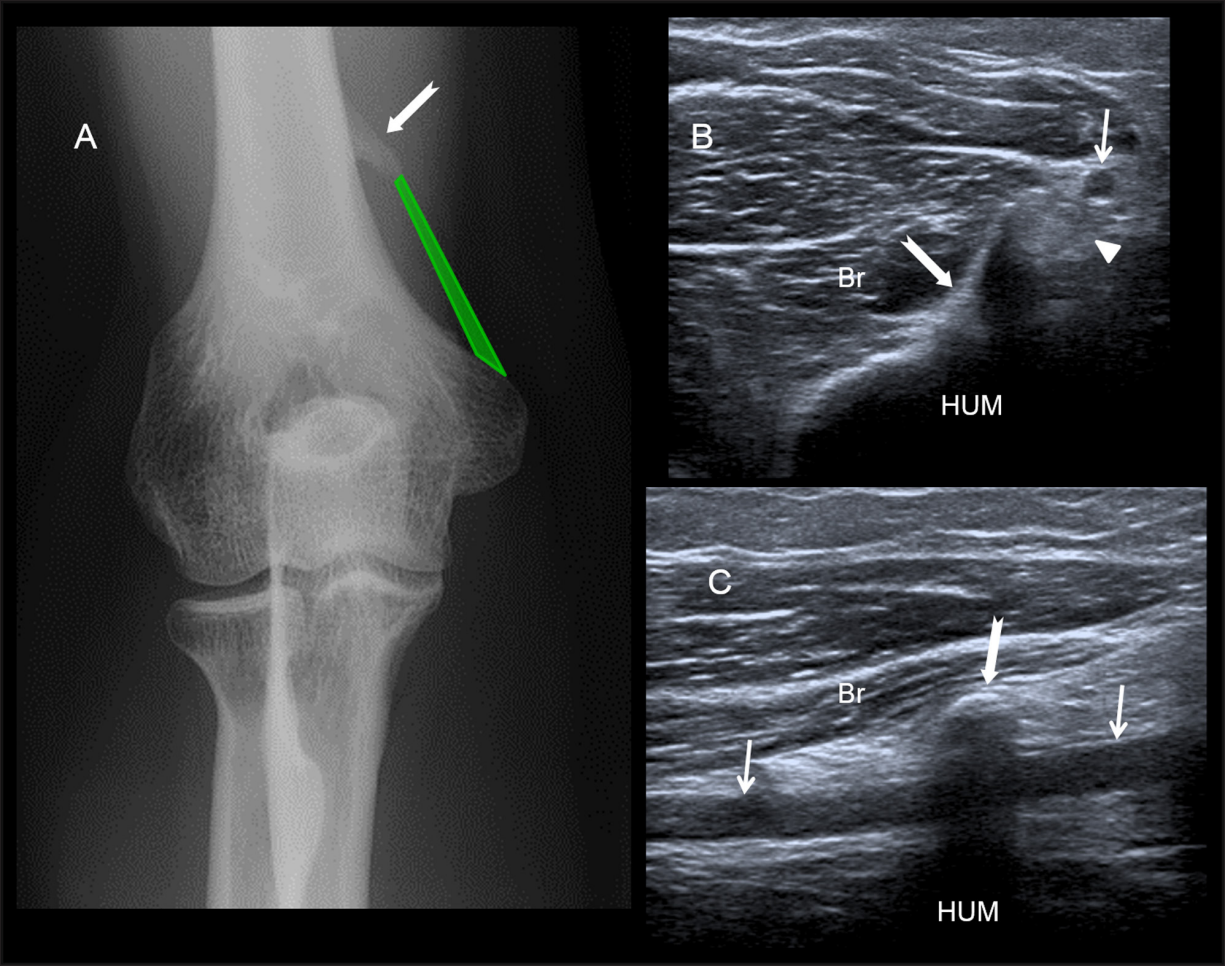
Supracondylar process on US examination. A) Posteroanterior radiographic view and US examination both on axial B) and longitudinal C) views. Br = Brachialis muscle, HUM = Humeral diaphysis, B = Biceps muscle. The bony spur is located anteromedially at the distal humerus. The ligament of Struthers (in green) connects the supracondylar process (white arrow) to the medial epicondyle, encasing neurovascular structures including the brachial artery (small white arrows) and the median nerve (white arrowhead) which may be entrapped.
